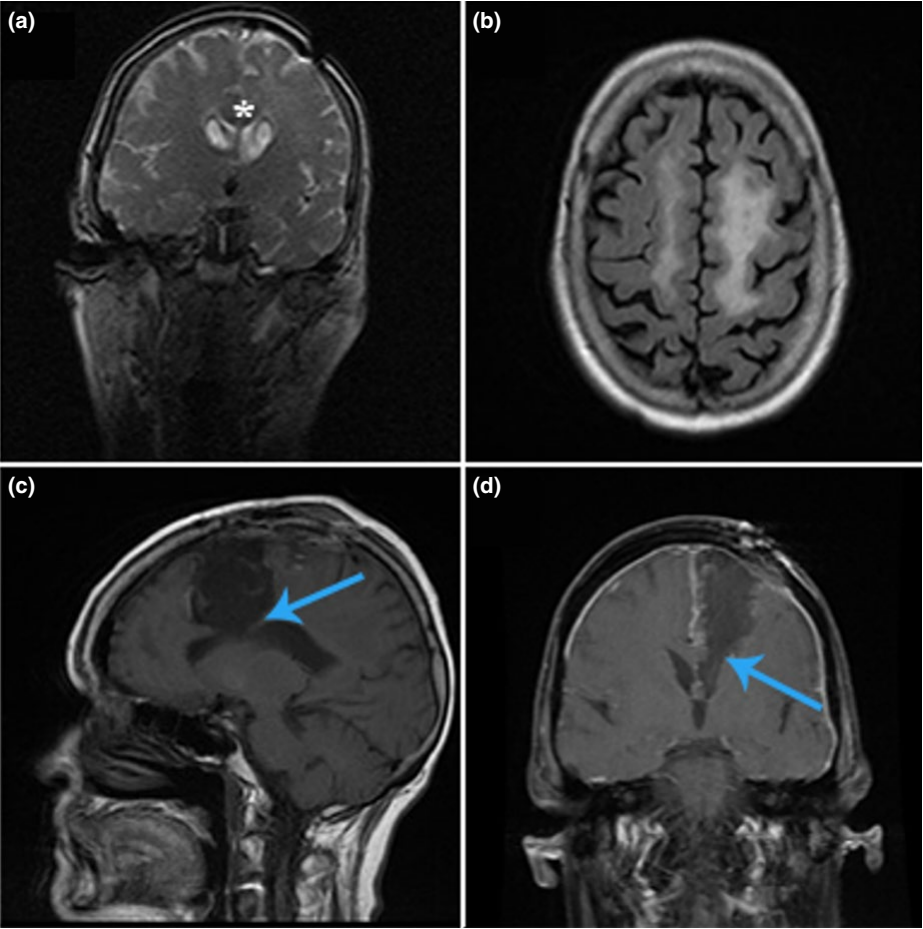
Permanent supplementary motor area (SMA) syndrome, case 1. Patient with a low‐grade glioma of the left frontoparietal region, hyperintensities seen on coronal T2 (a) and on sagittal T2 FLAIR (b) imaging. Postresection of tumor revealing dissection through the SMA into the corpus callosum (CC) on T1 with (d) and without (c) contrast (blue arrows). Asterisks in (a) designate location of CC
